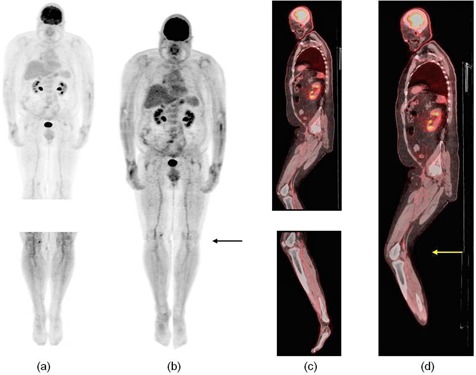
Patient 1: PET MIP images of (a) two body segments and (b) stitched image; fused PET/CT images of (c) two body segments and (d) stitched image.
